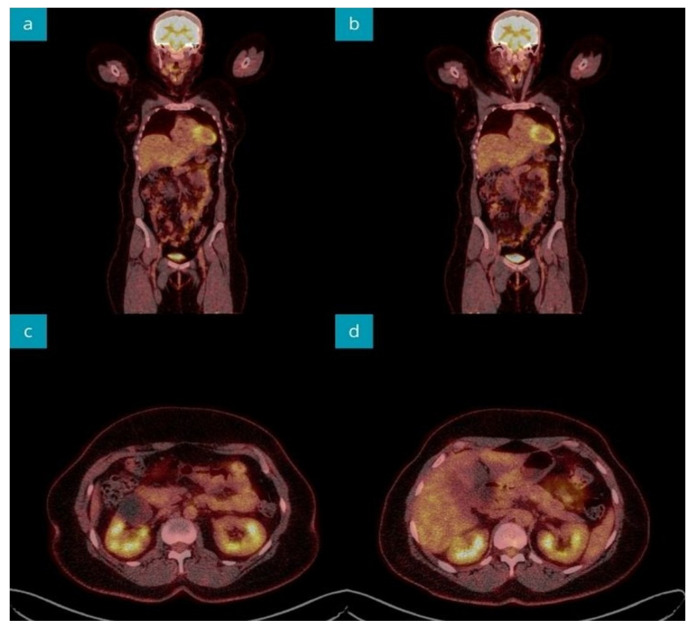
^18^F-FDG PET-CT imaging during the primary diagnostic process revealed abdominal organs without pathological ^18^F-FDG uptake. Coronal sections (a,b). Axial sections of pancreatic head (c), body, and tail (d).
